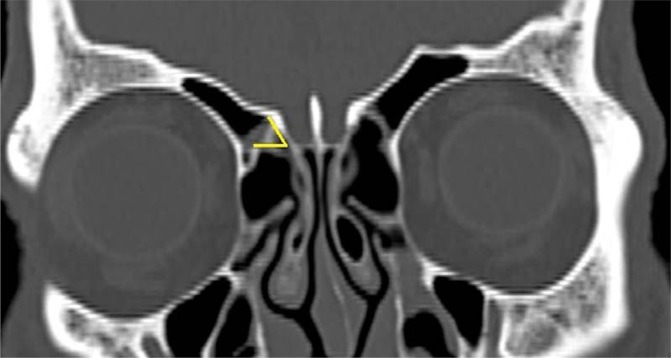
Gera classification^9^ was assessed by measuring at the angle formed by the lateral lamella of the cribriform plate and the continuation of the horizontal plane passing through the cribriform plate in the CT coronal plane and classified as Class I, II and III.
